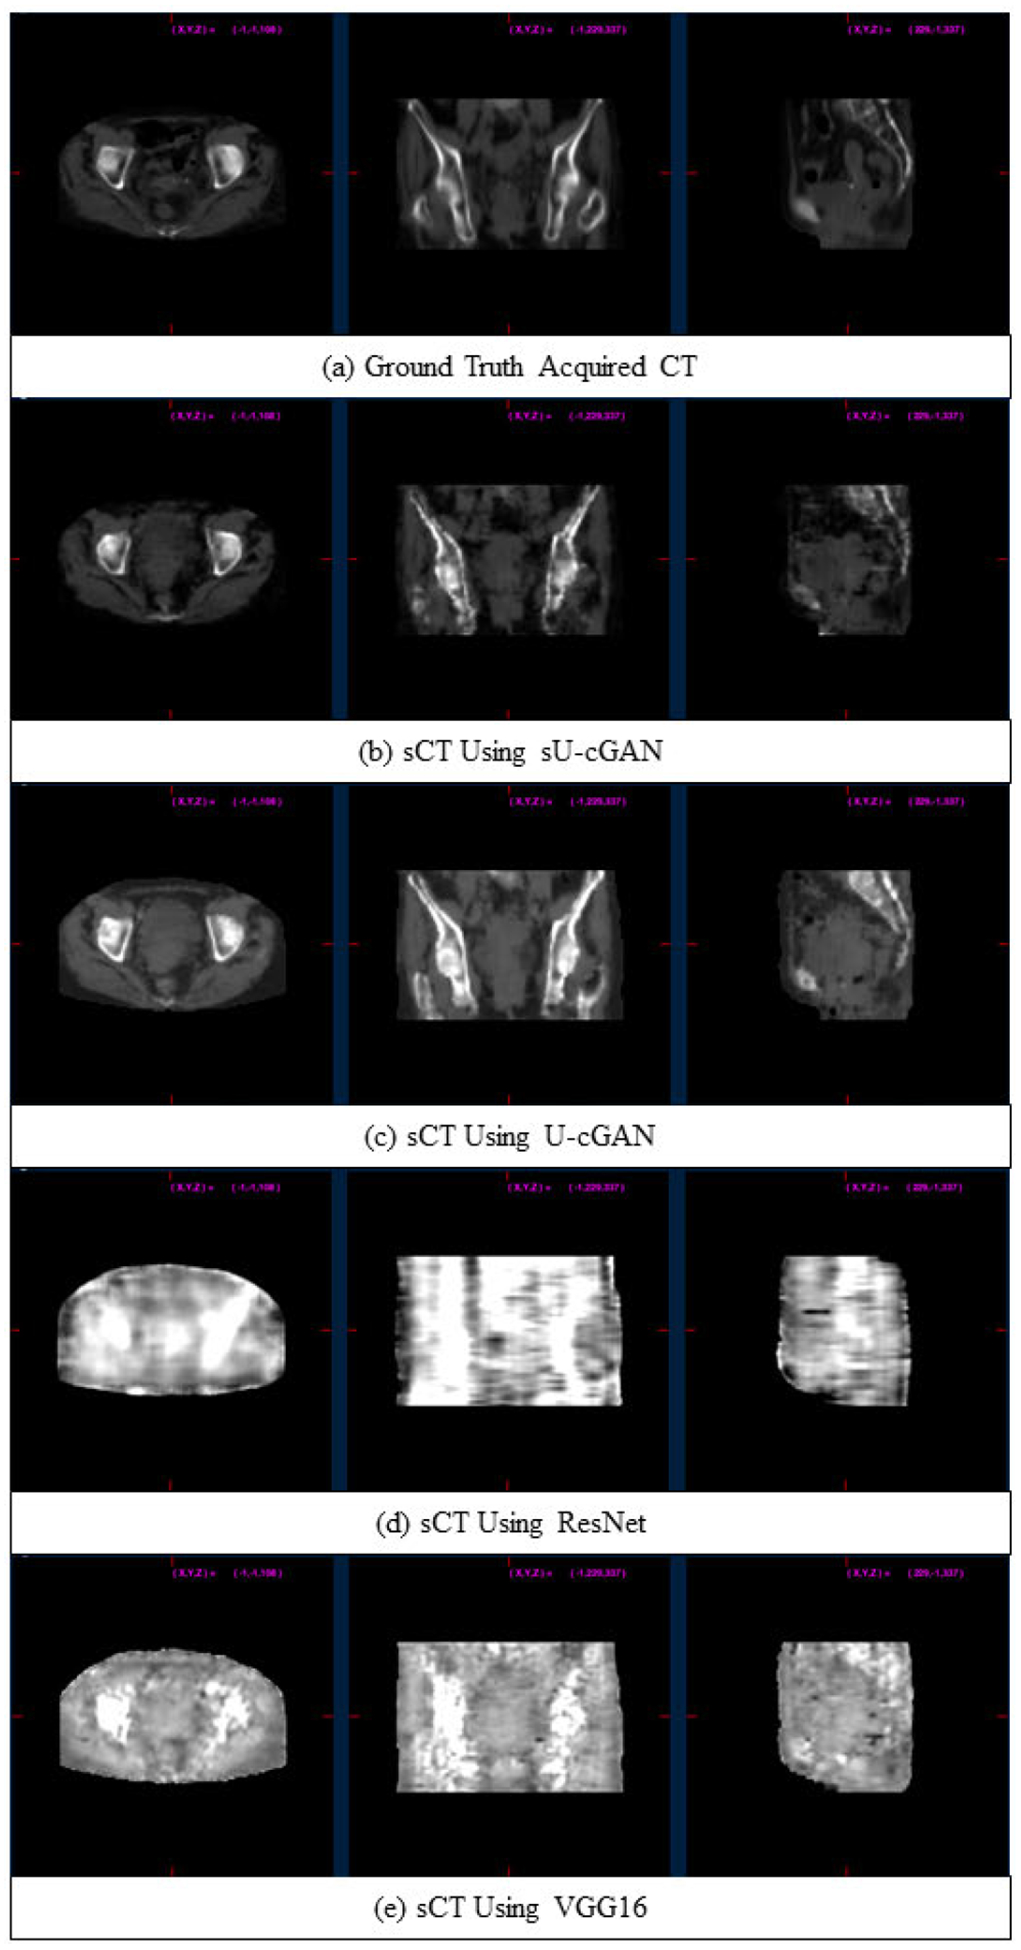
Axial, coronal, and sagittal (left to right) views of the measured CT (a), sCT using sU-cGAN (b), sCT using U-cGAN (c), sCT using ResNet (d), and sCT using VGG16 (e).
